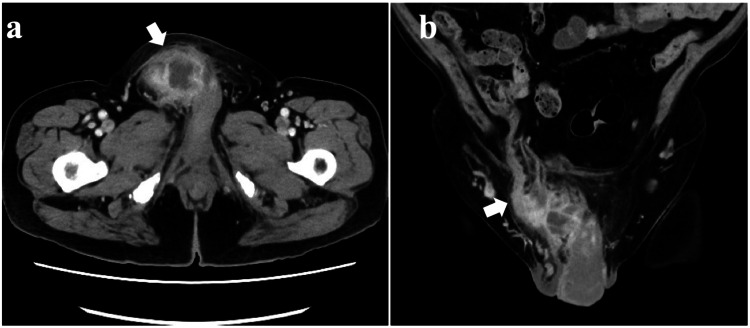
Abdominal contrast-enhanced CT on admission: (a) axial view and (b) coronal view. The abdominal CT demonstrates heterogeneous fluid collection within the right inguinal canal (a; arrow) and a thickened appendix within the hernia sac (b; arrow). CT: computed tomography.
